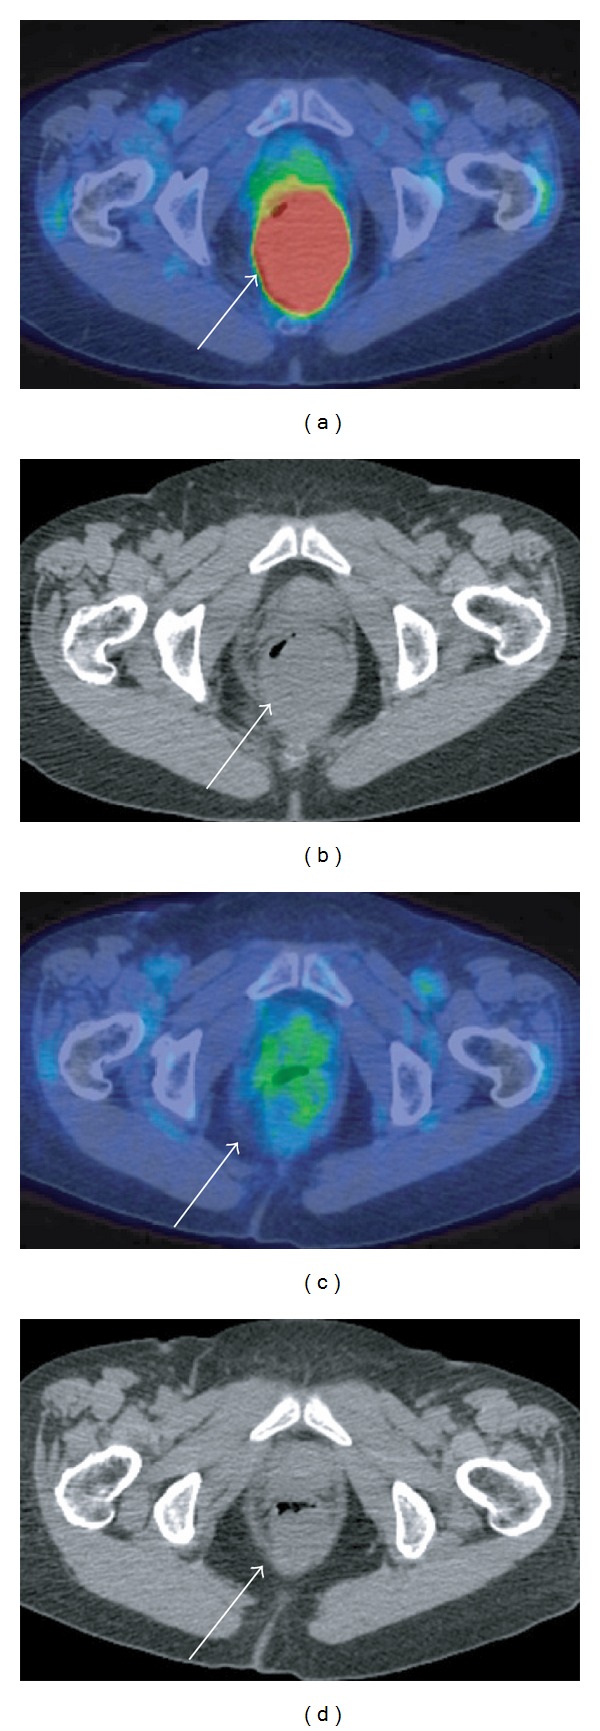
^18^FDG-PET/CT imaging prior to Ima treatment (a, b) and 1 month after treatment (c, d).
